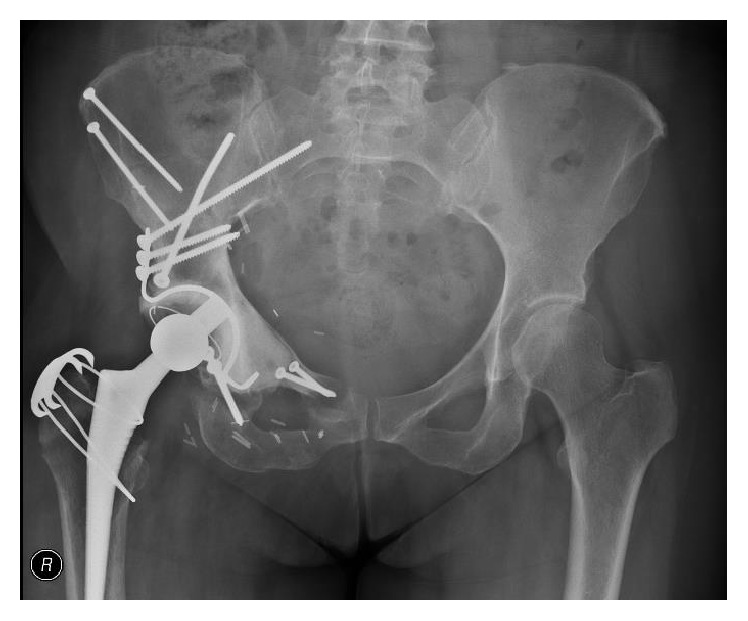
Postoperative X-ray at 6 months. Asymptomatic nonunion is seen at the ischial and pubic osteotomies. The configuration of screws and plates and screws through flanged acetabular cages was variable between cases.
